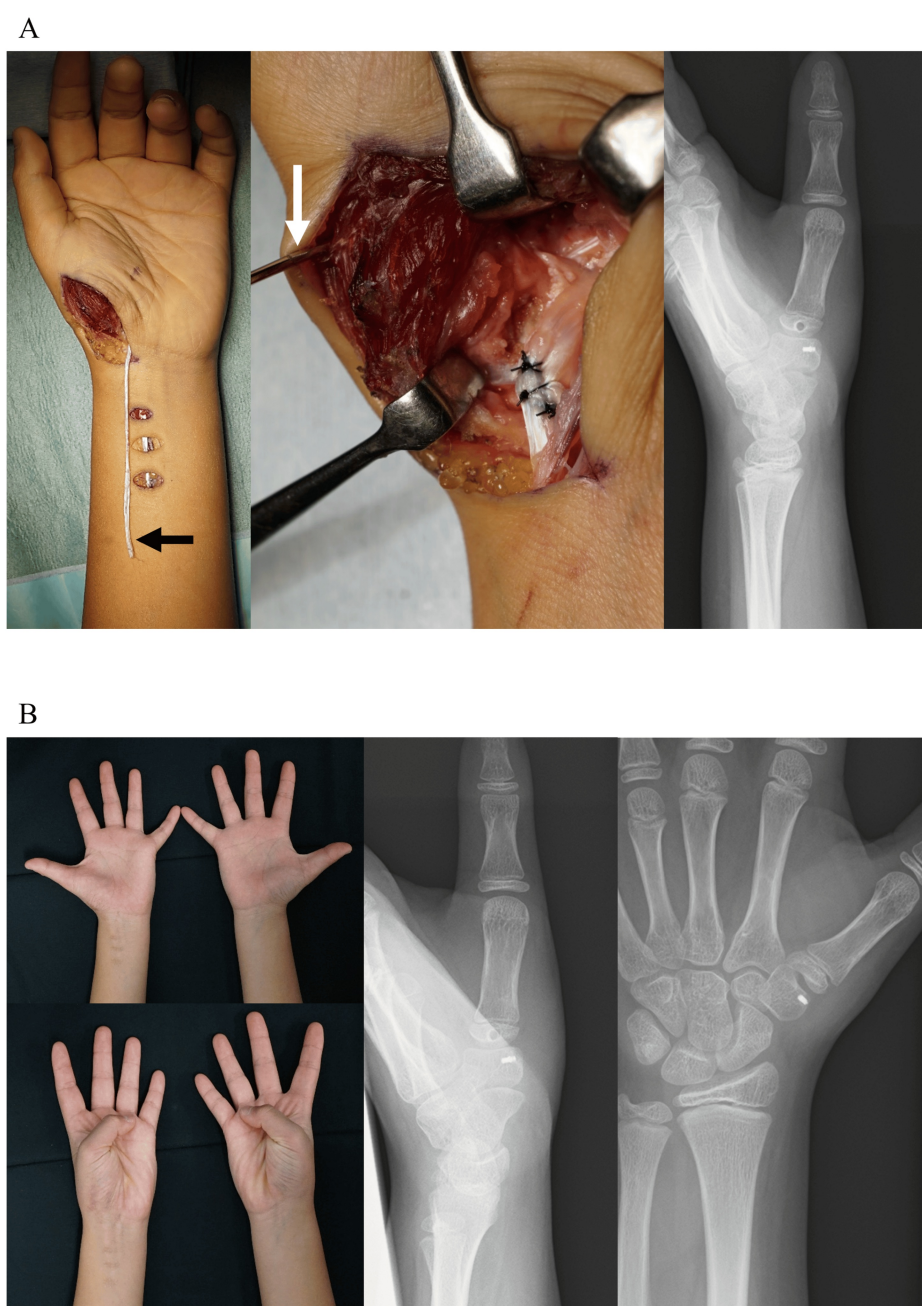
Images at and after the secondary surgery. (A) Intraoperative finding of secondary Eaton-Littler’s ligament reconstruction. The black arrow shows a half slip of the flexor carpi radialis tendon. The white arrow shows a guide wire for drilling from the volar to the dorsal region at the base of the first metacarpal while preserving the growth plate. A postoperative radiograph shows the reduced carpometacarpal (CMC) joint of the left thumb with internal stabilization using a half-slip of the flexor carpi radialis. (B) Fourteen months after secondary ligament reconstruction surgery. Full thumb range of motion of the left hand compared with the right hand. The left thumb CMC joint is completely stable in the reduced position on X-ray images.
